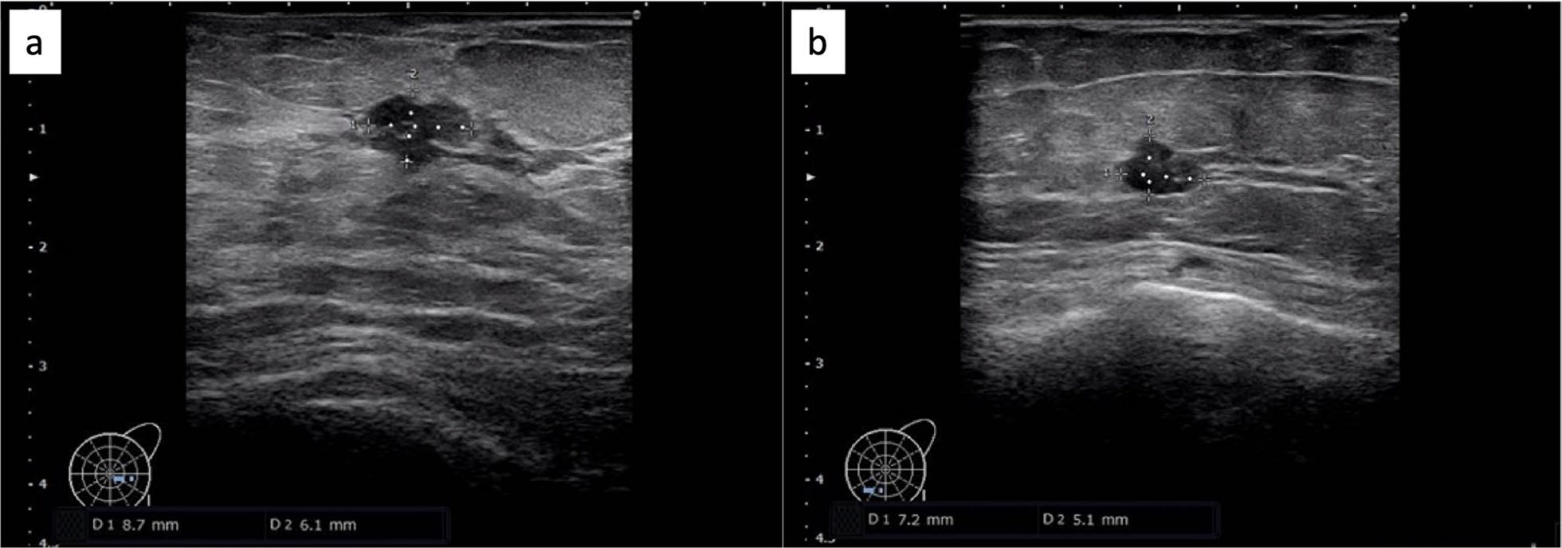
Breast ultrasound findings. a 4 × 3 mm oval, well-defined, smooth, hypoechoic mass in the left lower outer region of the left breast (Category 3). b 6 × 6 mm round, well-defined, smooth, hypoechoic mass in the left lower inner region of the left breast (Category 4)
